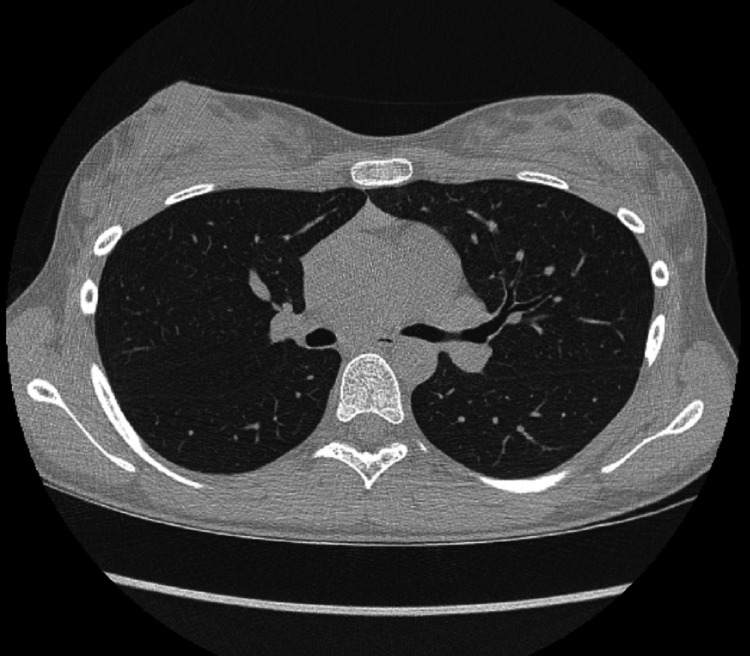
High-resolution computed tomography scan showing normal lung interstitium and parenchyma.
